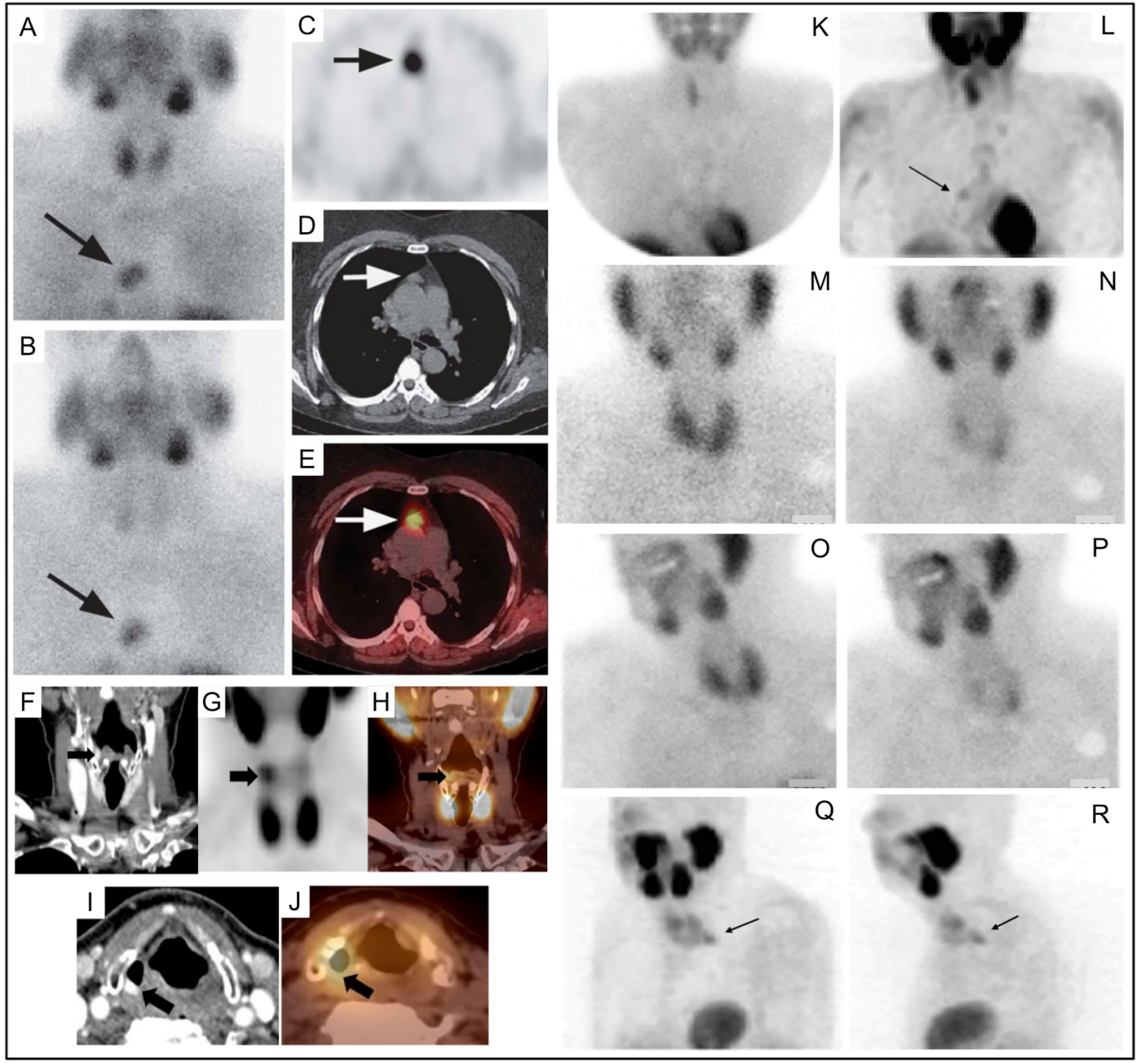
Parathyroid planar imaging should include the mediastinum, where a large percentage of ectopic PA occur. Planar scintigraphy of an ectopic PA (A) 20min and (B) delayed 2h. ^99m^Tc-sestamibi SPECT/CT imaging can aid in anatomical localization and is particularly helpful for ectopic PA. ^99m^Tc-sestamibi SPECT/CT of mediastinal PA on axial SPECT [(C)-black arrow], axial CT [(D)-white arrow], and axial fused SPECT/CT [(E)-white arrow]. ^99m^Tc-sestamibi SPECT/CT of an ectopic PA in a particularly rare position near the right piriform sinus on coronal CECT [(F)-black arrow], coronal SPECT [(G)-black arrow], coronal fused SPECT/CT [(H)-black arrow], axial CECT [(I)-black arrow], axial fused SPECT/CT [(J)-black arrow]. SPECT imaging can also be helpful when planar imaging is negative (K, M, N) as in (L) showing mediastinal PA (arrow) adjacent to the cardiac border. Another PA behind the left lobe of the thyroid was not identified on planar anterior early 99mTc-sestamibi (M), delayed 99mTc-sestamibi (N), or on planar oblique images (O, P), but was identified on SPECT [(Q, R), arrows]. Reproduced with permission from: (A–E)
